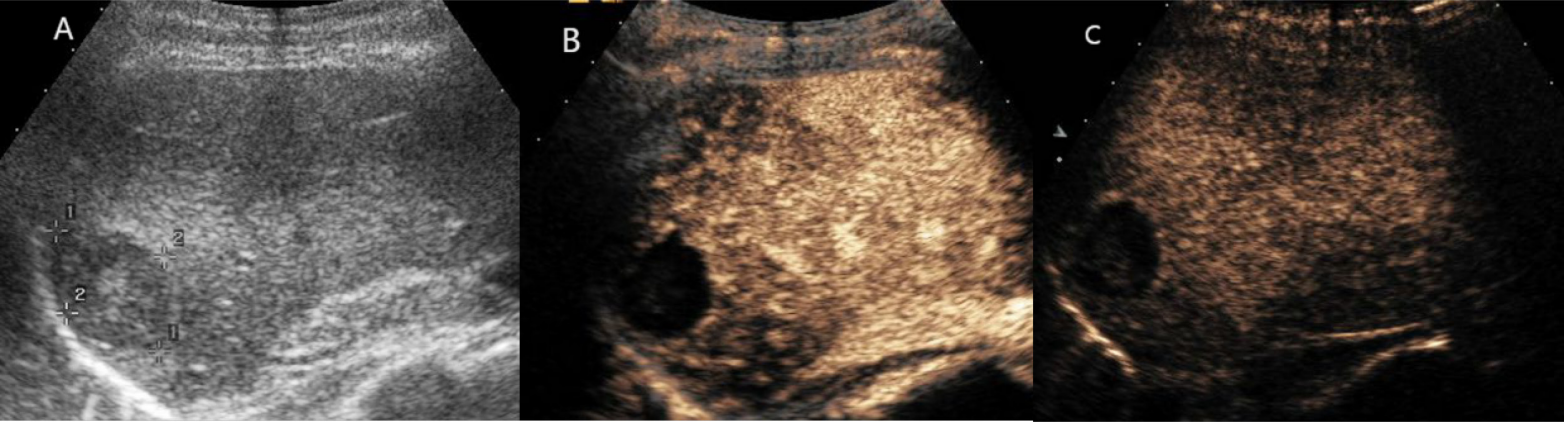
Hepatic hemangioma showed peripheral circular enhancement in all vascular phases (A) Ultrasound revealed a hypoechoic mass in the right liver. (B) and (C) CEUS showed peripheral circular enhancement throughout the whole vascular period.
